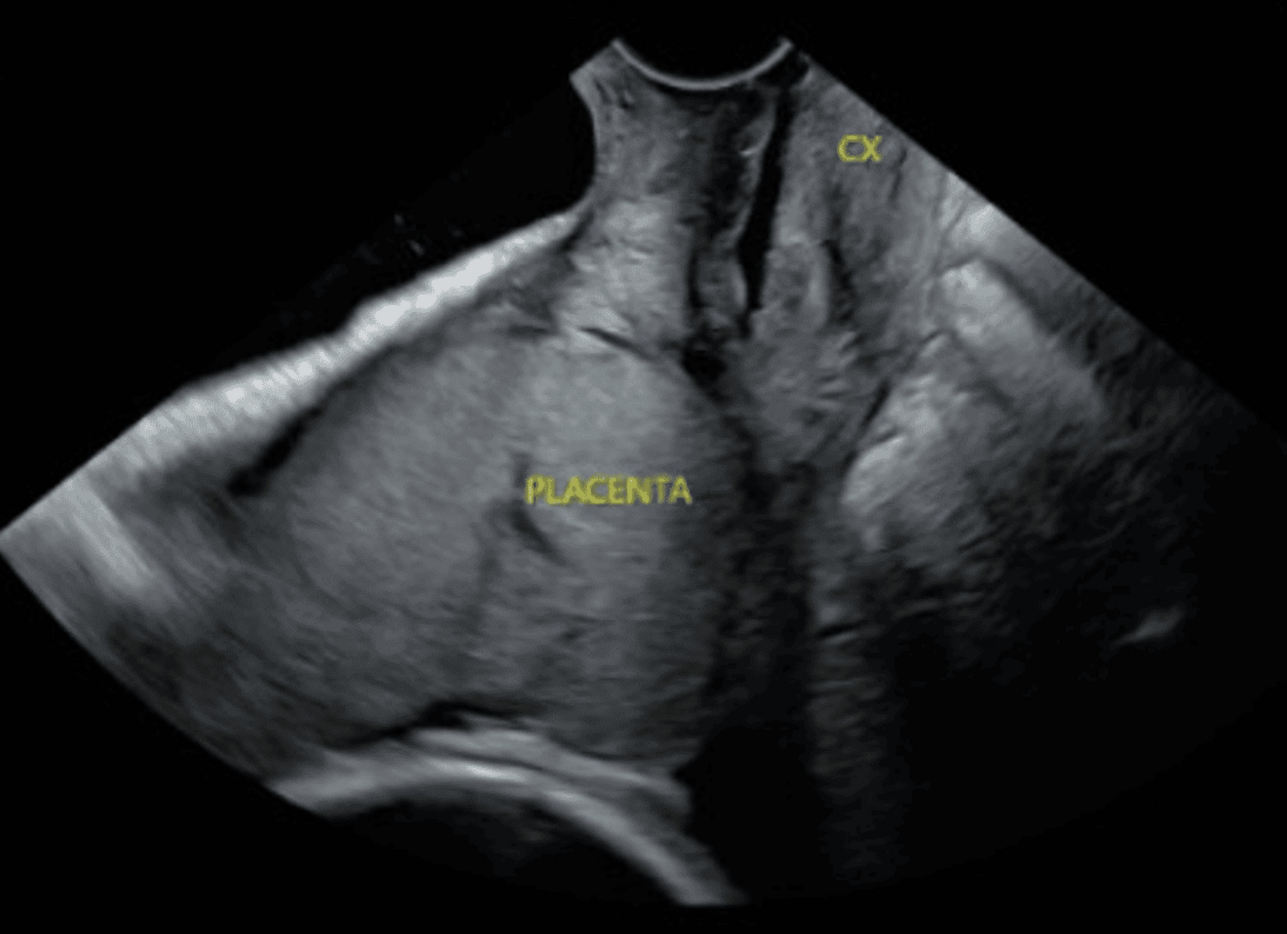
Ultrasound image showing the placenta completely covering the internal cervical os
